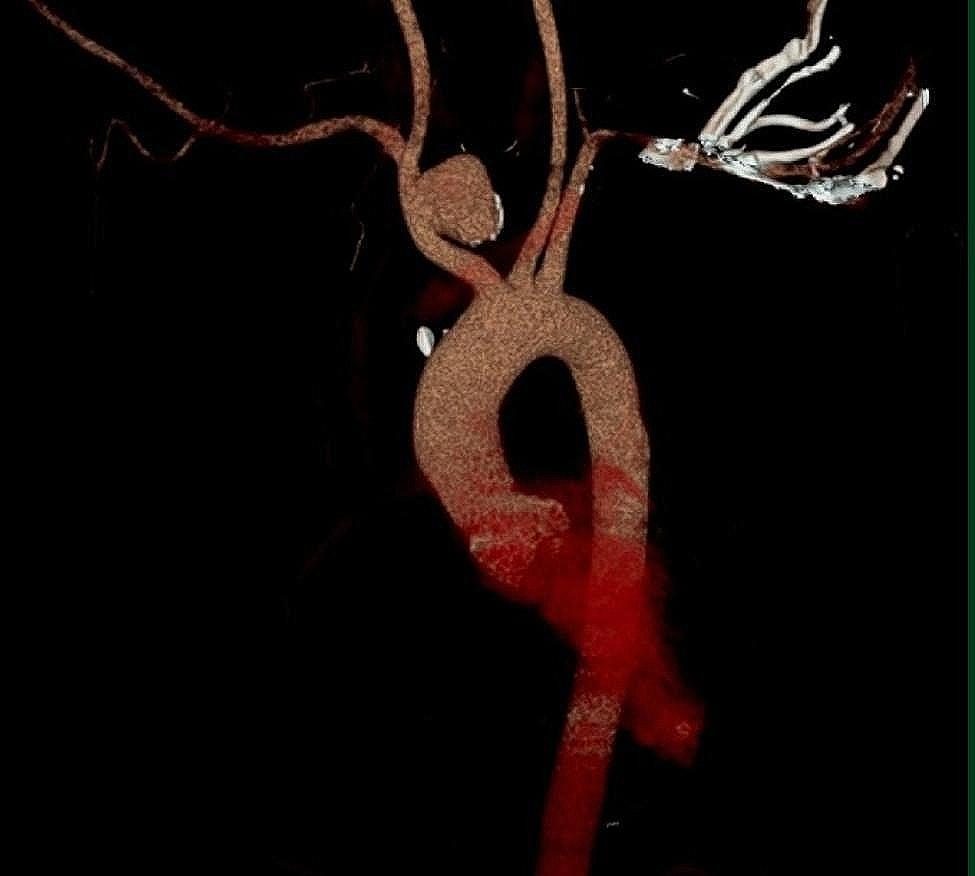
Preoperative CTA imaging and three-dimensional reconstruction revealed a 3.6*2.4 cm saccular aneurysm of the innominate artery that involved the right proximal subclavian and common carotid arteries
